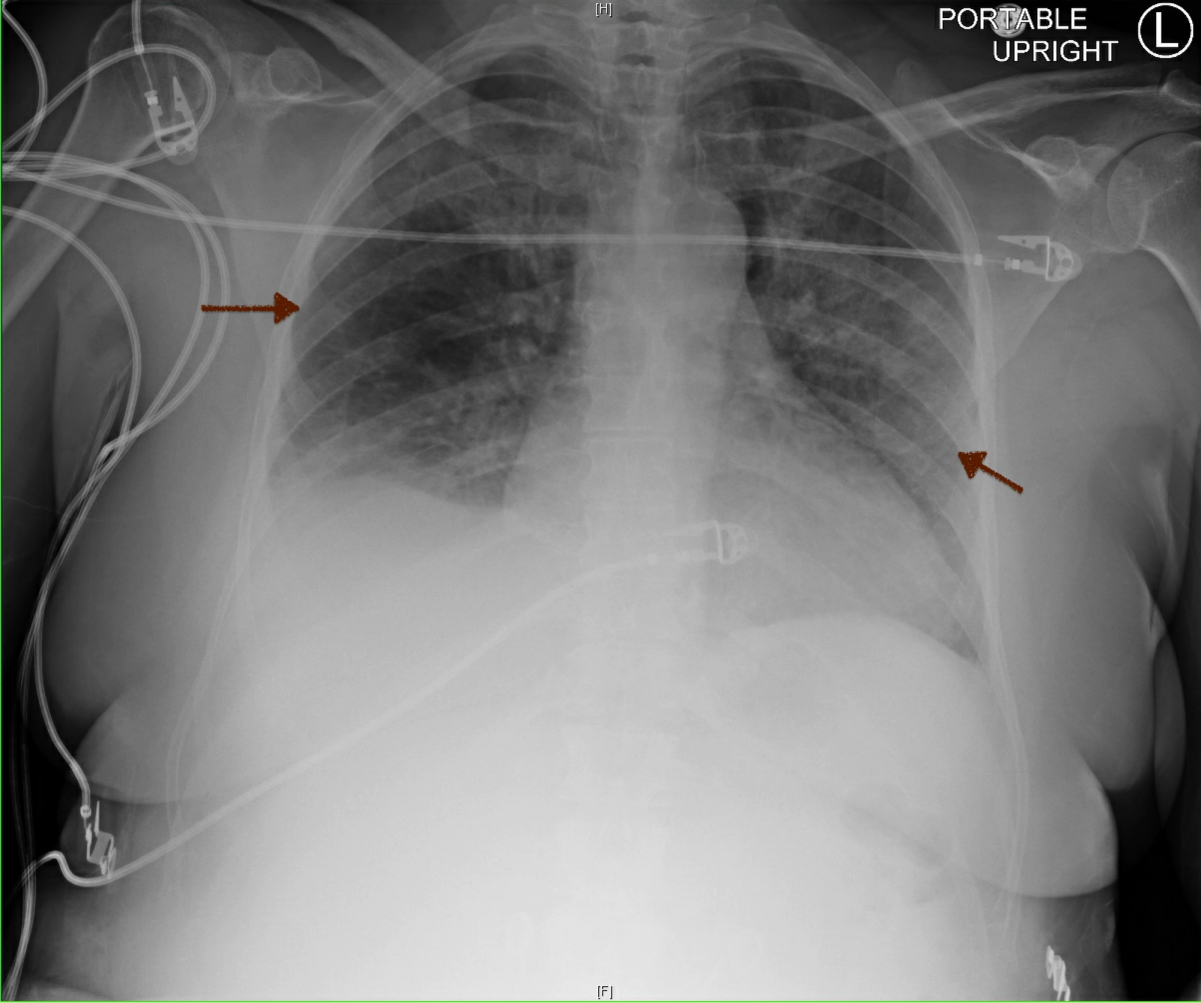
Chest X-Ray Interval extubation along with gradual improvement of airspace opacities (red arrows). L: Left. H: Head. F: Feet.
